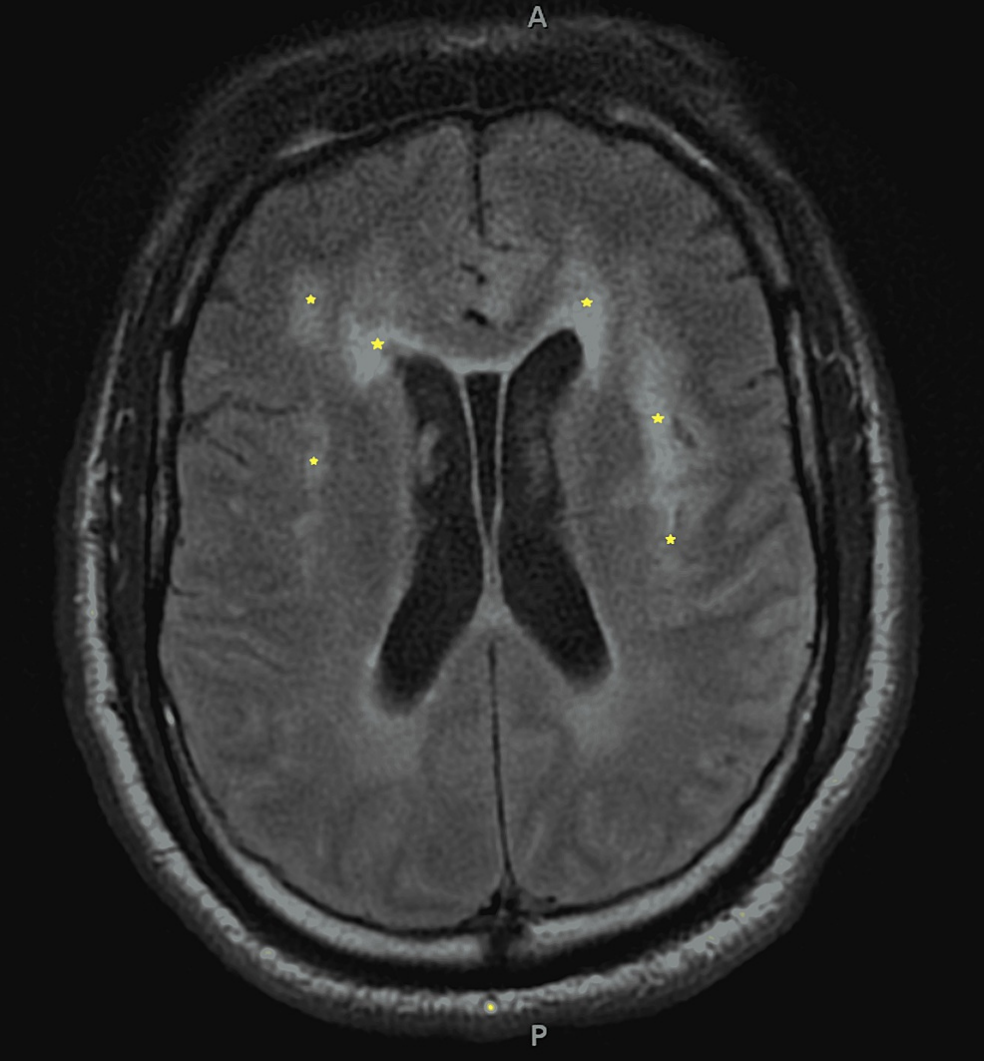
Axial T2 FLAIR MRI Brain prior to hemorrhagic conversion *Multiple areas of bilateral restricted diffusion involving the medial left anterior frontal lobe, left parietotemporal lobe and bilateral insula suspicious for encephalitis
